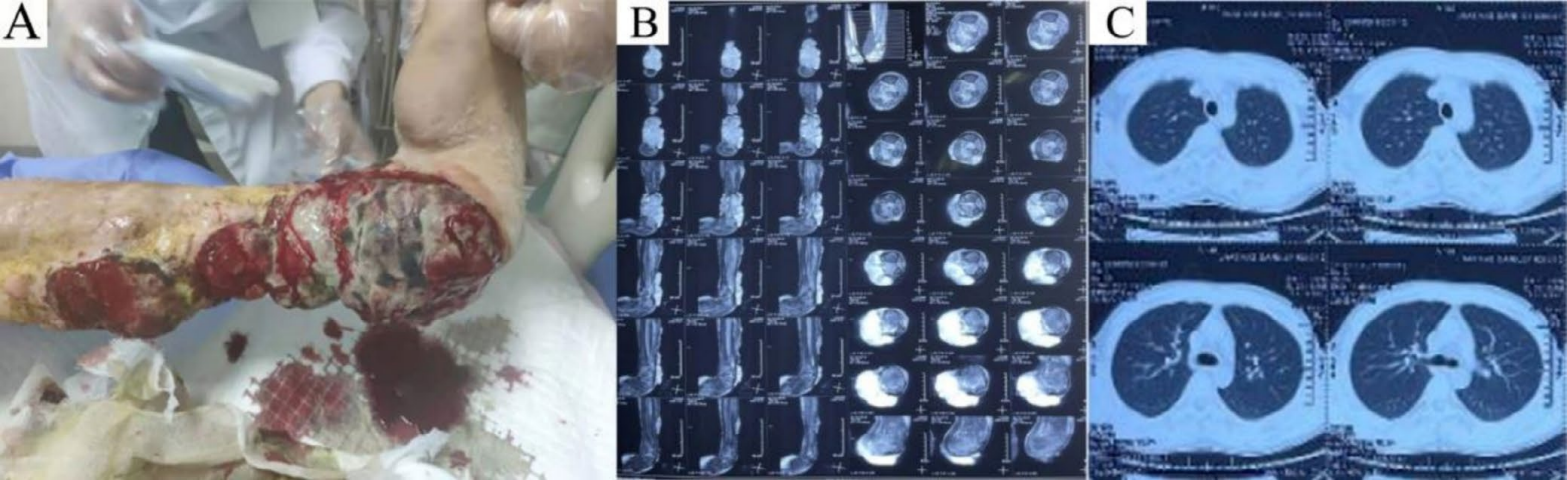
Pre‐amputation clinical and imaging features of the left lower limb. (A) Preoperative skin lesions on the left lower limb. (B) Preoperative MRI of the left lower limb. (C) Preoperative non‐contrast CT scan of the lungs.
